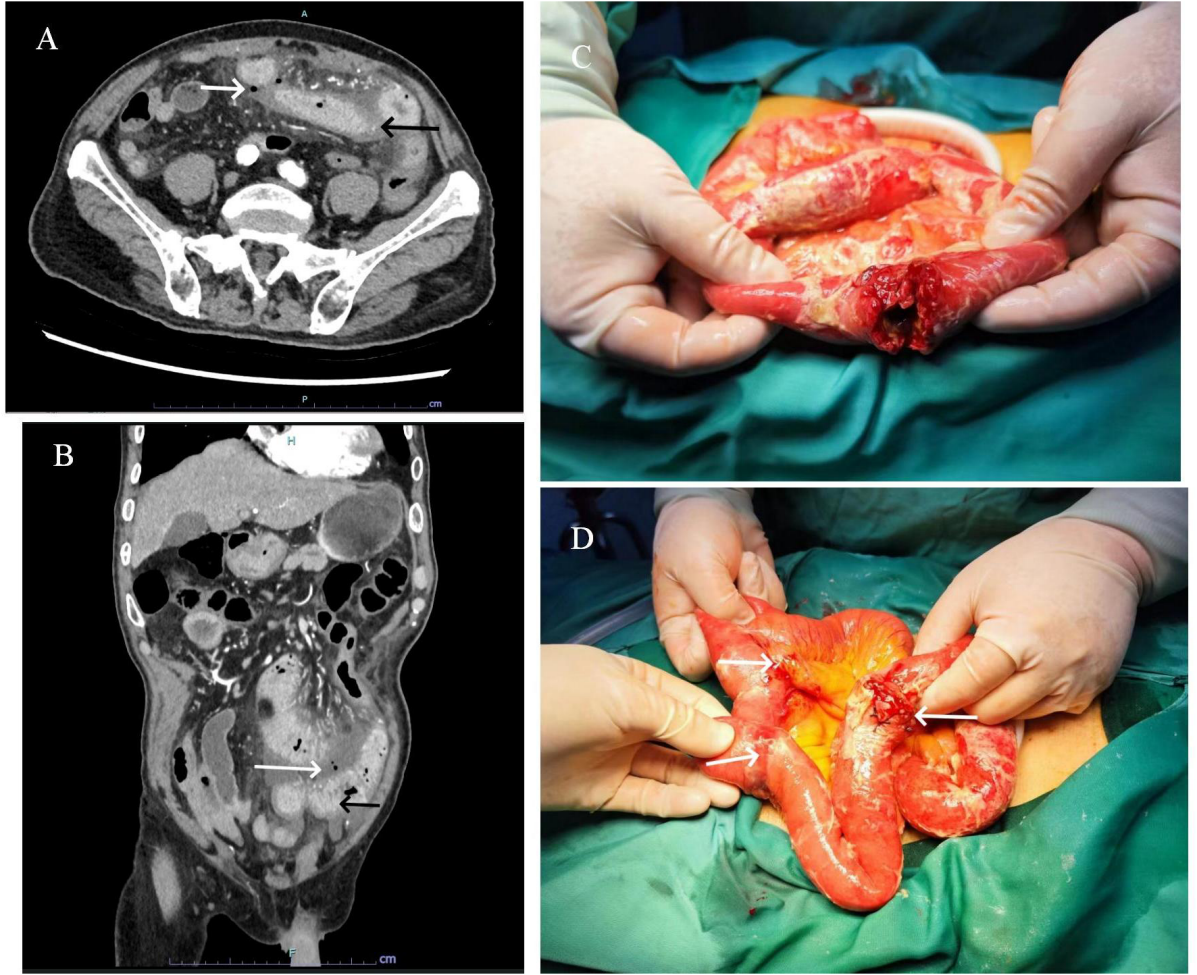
Transverse (A) and sagittal (B) enhanced CT images of the abdomen reveal a thickened, abnormally enhanced bowel wall (black arrows) and free air bubbles in the mesenteric space (white arrows). A cauliflower-shaped mass with a central perforation was identified in the proximal small intestine (C). Additionally, two sclerotic nodules were observed distally, invading the plasma membrane layer (D).
